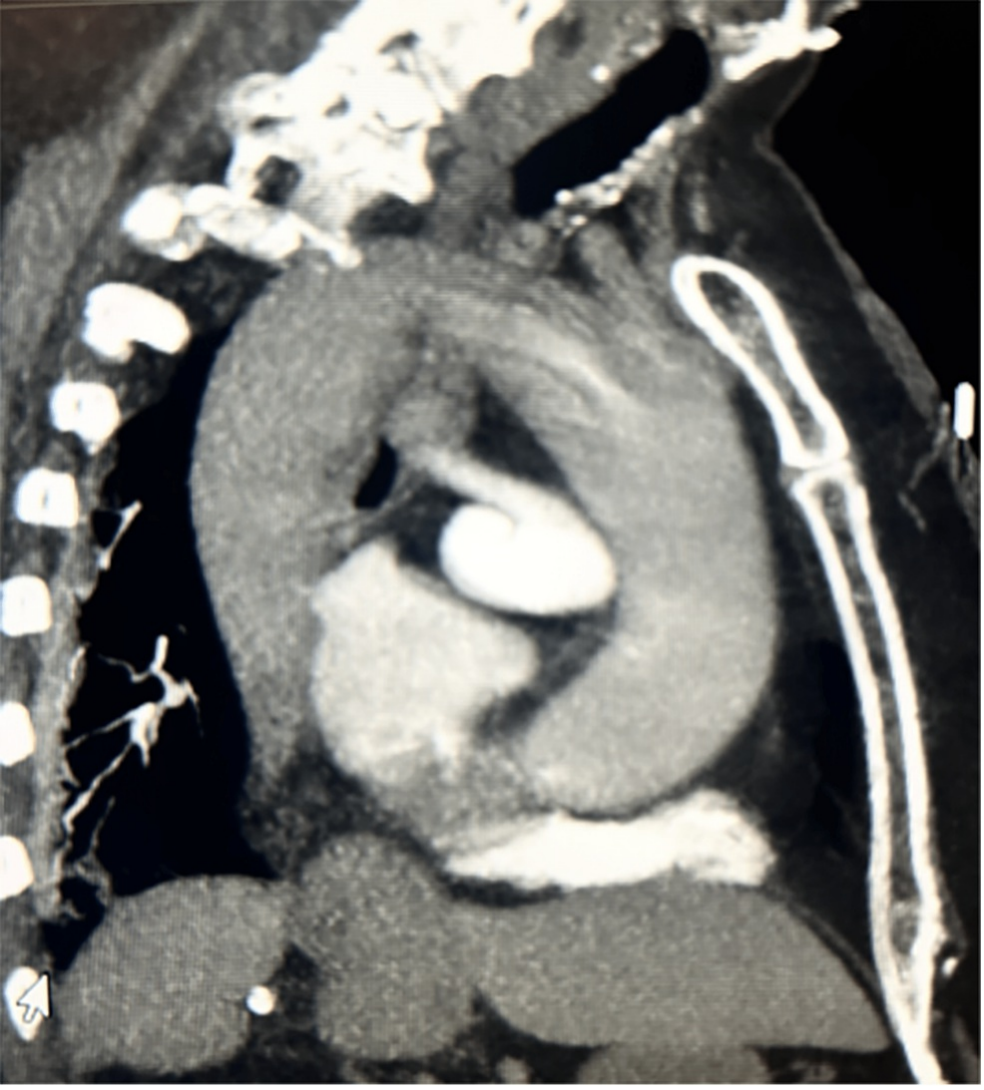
A CT chest with contrast scan demonstrates an elongated aorta. CT: Computed Tomography
